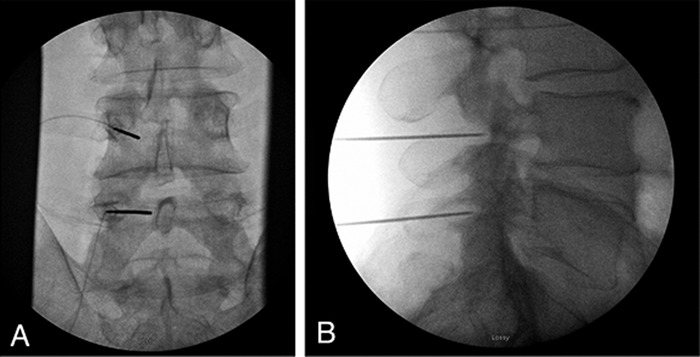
(A) Anterior posterior and (B) lateral radiographic images of the introducer placement for the SPRINT peripheral nerve stimulation device at the level of L4 and L5 on the left side, targeting the median nerves for the patient described in case 1.
